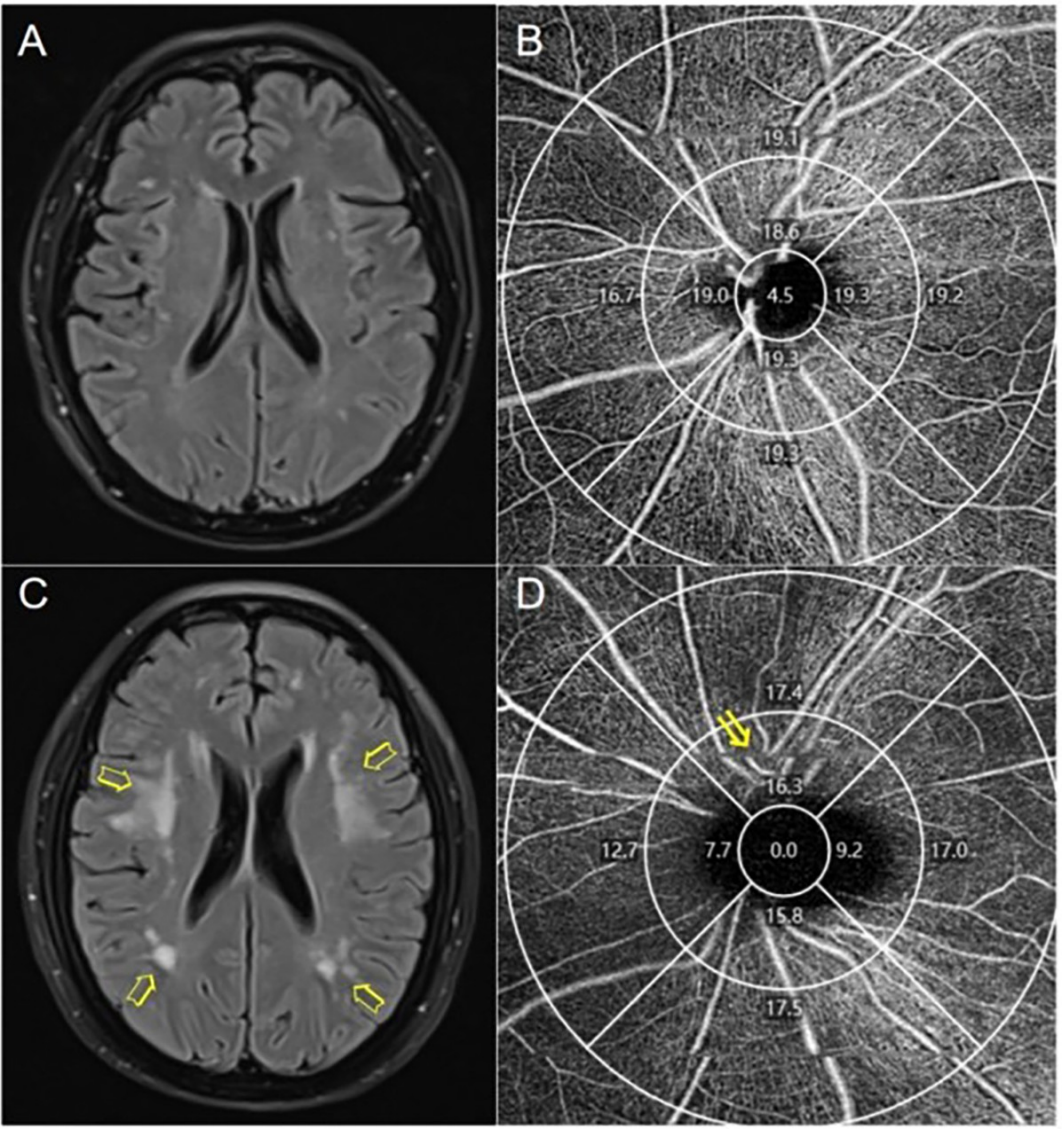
Representative images of OCT-A and brain MRI from study participants. (A) Mild white matter lesions (Fazekas scale score = 1, Scheltens scale score = 7). (B) Peripapillary vessel density (VD) of a patient with mild white matter lesions. (C) Severe white matter lesions (Fazekas scale score = 6, Scheltens scale score = 25). (D) Patient with severe WMH (arrows) showing decreased peripapillary vascular density in the superior quadrant of the inner ring (arrows).
